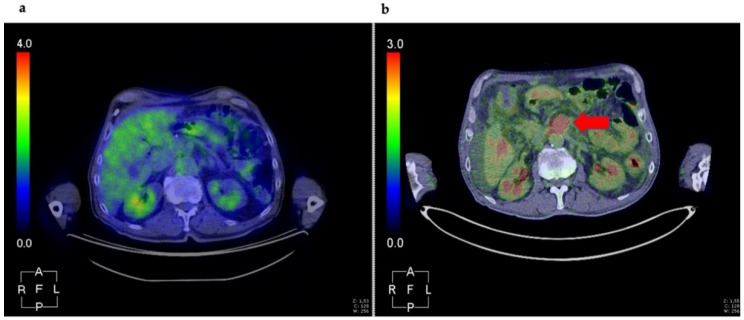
Patient diagnosed with PC (head), received six cycles of NAT (May–October 2019) and subsequent resection (December 2019). First PET/CT evaluation was performed in July 2020 for suspicious local relapse. The result was negative (a). During follow-up, in June 2021, a second scan revealed a preaortic mass (red arrow) compatible with disease relapse (b). Figure is property of the authors.
